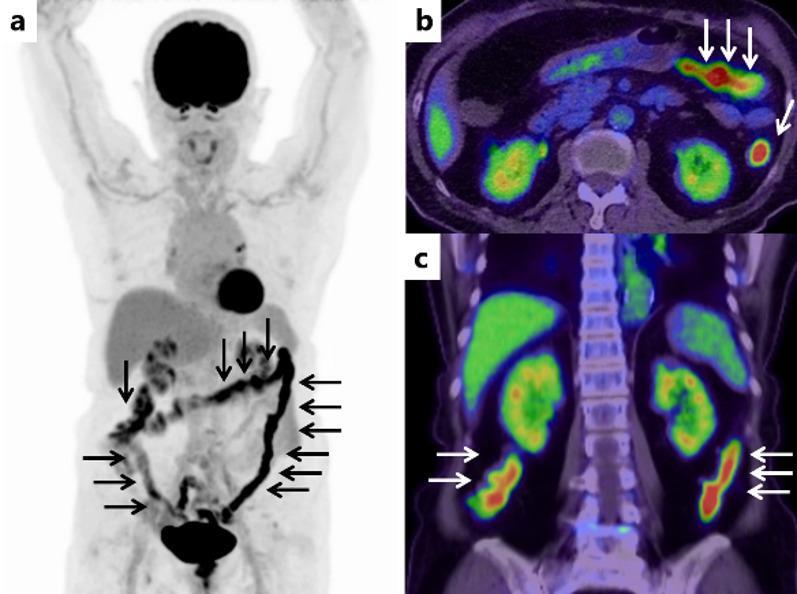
Metformin-related bowel FDG uptake. a FDG-PET/CT (MIP image), b FDG-PET/CT (fusion image) (axial image), (c) FDG-PET/CT (fusion image) (coronal image). A man in his 80s with type 2 DM treated with metformin presented without any symptoms. FDG-PET/CT showing diffuse uptake throughout the colon and distal small intestine (SUVmax: 6.8) (a–c; arrow). No bowel wall thickening or inflammation was observed in the FDG-positive bowel (data not shown). Abbreviations: FDG-PET/CT, F-fluorodeoxyglucose positron emission tomography/computed tomography; FDG, F-fluorodeoxyglucose; DM, diabetes mellitus
